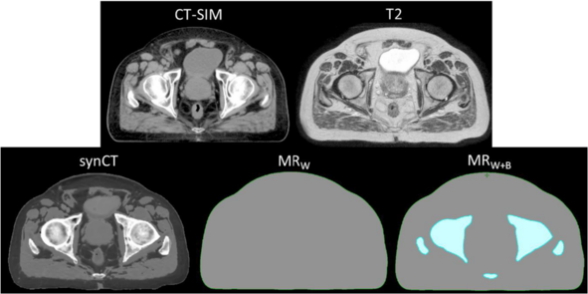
CT and MR-derived CT surrogate images. (Top) Patient CT and T2-weighted MR images. (Bottom) MR-derived CT surrogates used for study: synthetic CT (synCT), T2 with water bulk density assignment (MR_W_), T2 with bulk density assignments of water and bone (MR_W+B_)
